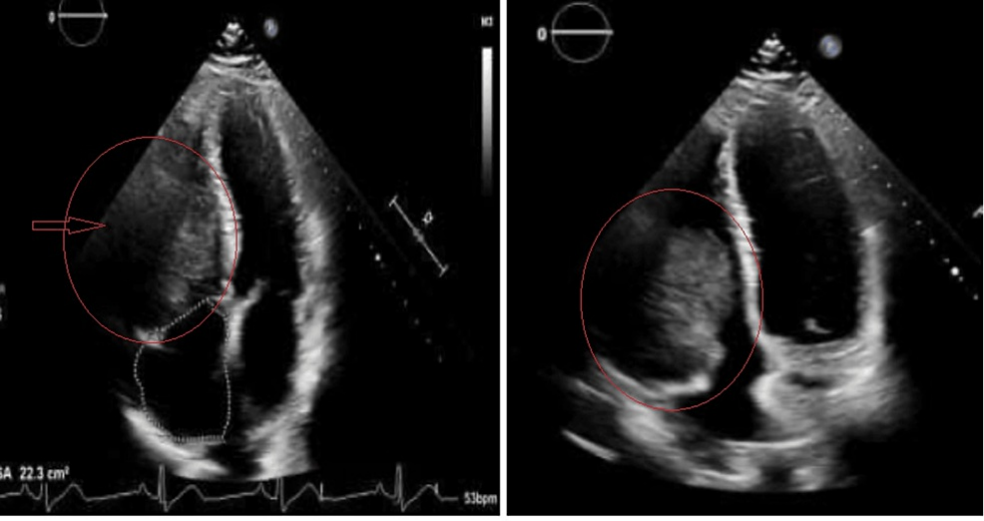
Echocardiogram showing a right ventricular mass
